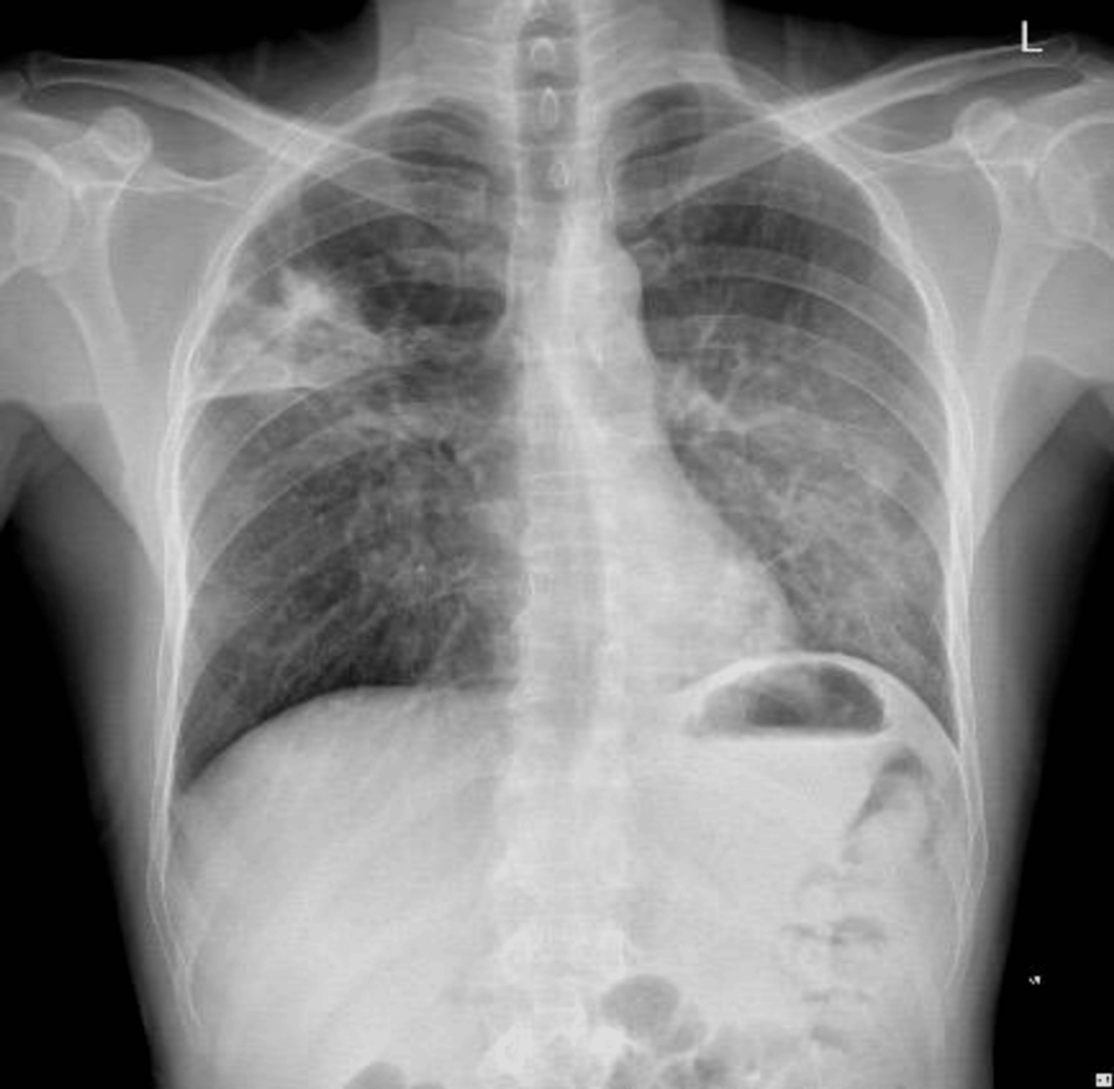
Chest X-ray on discharge shows improvement of Nocardia otitidiscaviarum pneumonia.
